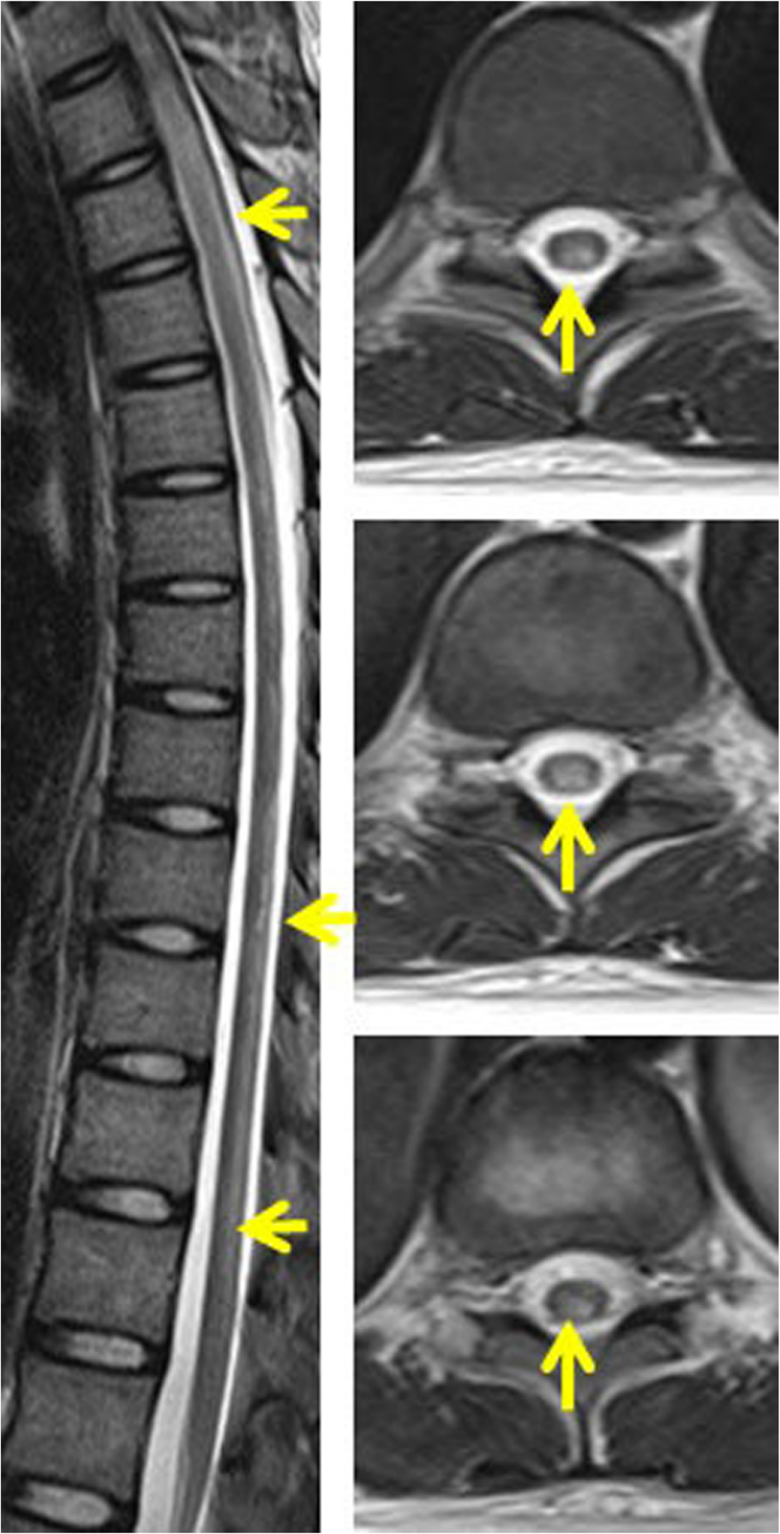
The MRI of spinal cord showed abnormal hyperintensities within the dorsal thoracic spinal cord
